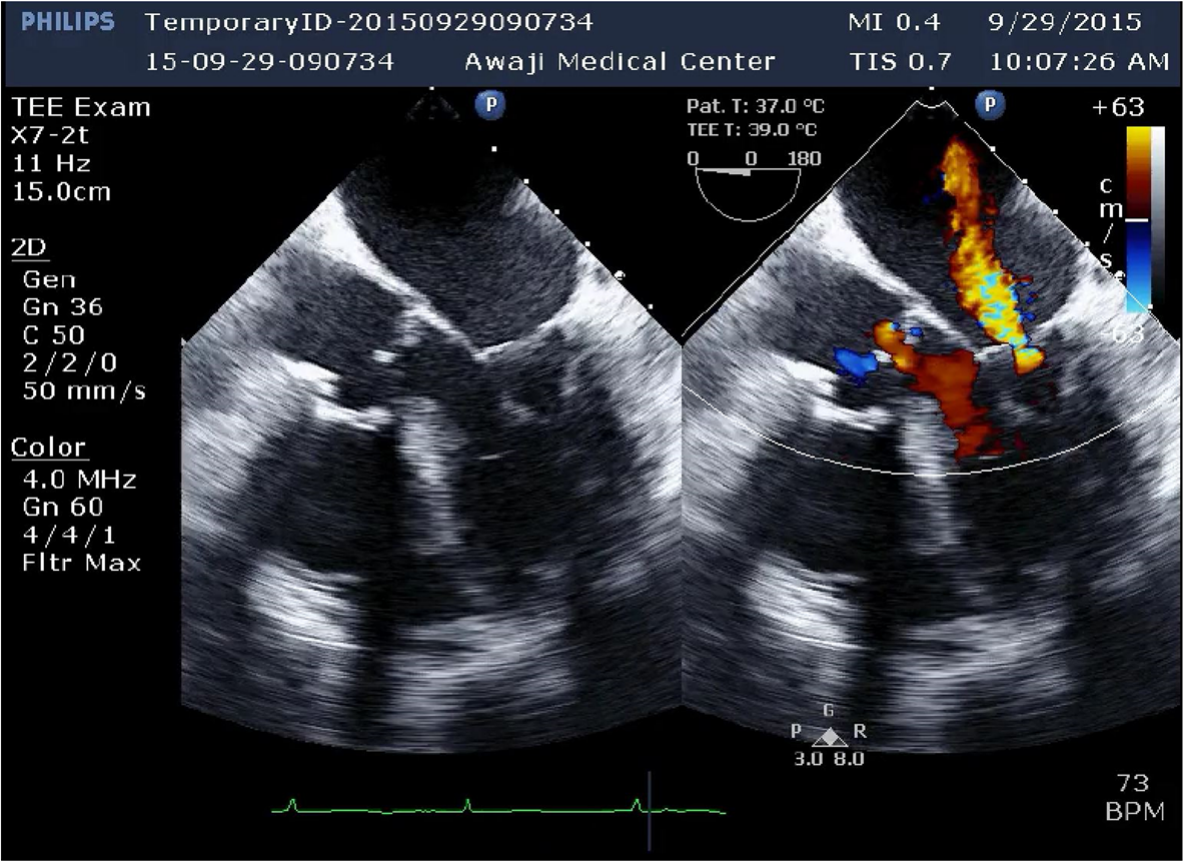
Preoperative transesophageal echocardiography images showing mitral valve and a color Doppler image showing mild to moderate functional mitral regurgitation
